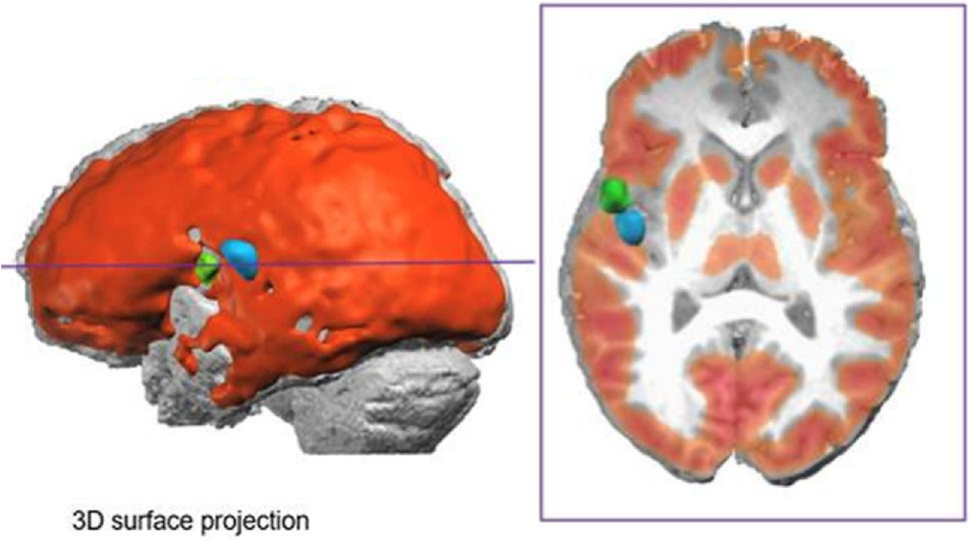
This 19 years old female ETLE patient was known for seizures since the age of 4. All three imaging modalities (PET, SPECT, ESI) were concordant for the insular region and the frontal and temporal opercular region. She was operated at the age of 7 years and resection resulted in an excellent outcome (ILAE I) after 12 years of follow-up. No phase II was carried out prior to surgery, due to complete concordance of the results. Blue: maximum perfusion of ictal SPECT, green: ESI, red: PET metabolism is found in red with an area of absent concordant with ESI and ictal SPECT. Left is left in this and all figures
